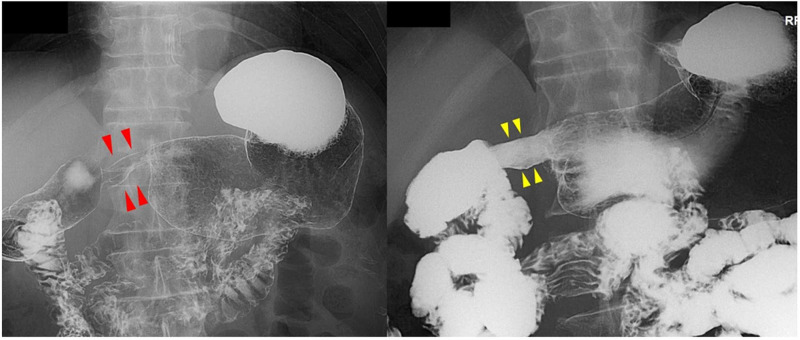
X-ray image showing tunnel-like narrowing of the gastric antral portion X-ray image showing tunnel-like narrowing of the gastric antral portion (left panel, the narrowed portion shown by red arrowhead). The luminal patency of the antral portion was relatively good due to insufflation (right panel, the narrowed portion shown by yellow arrowheads)
